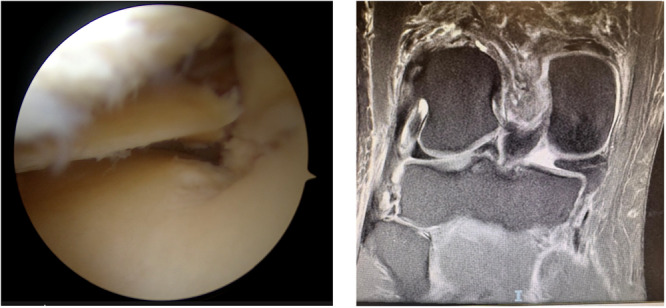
Arthroscopic view (left) showing a complete avulsion of the medial meniscus posterior root (MMPR). Coronal T2‐weighted magnetic resonance imaging (MRI) (right) demonstrates meniscal extrusion and disruption of the posterior root attachment, consistent with a MMPR tear.
